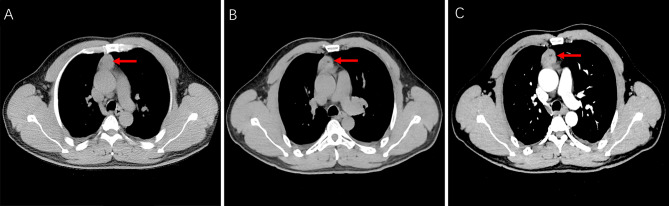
A plain chest CT scan showing a soft tissue mass in the anterior middle mediastinum, measuring approximately 2.6 cm × 2.8 cm×5.4 cm with patchy fatty component on July 28, 2023 (A); Plain and contrast-enhanced measuring 2.5 cm ×2.7 cm × 5.1 cm and showed a slight homogeneous enhancement after an intravenous contrast administration on August 14, (B, C)
